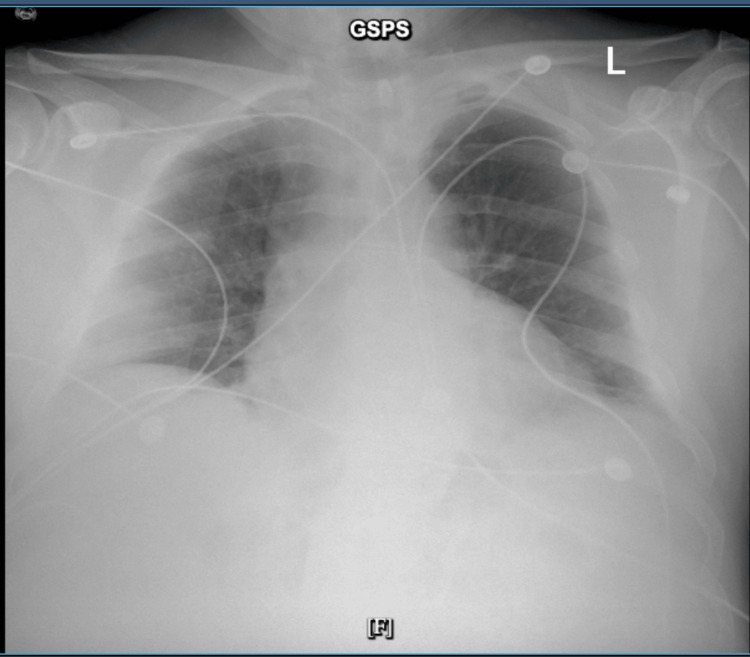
A chest radiograph demonstrated cardiomegaly and left basilar opacities. The image shows an enlarged cardiac silhouette and hazy opacities in the left lower lung zone, compatible with atelectasis or COVID-19-related pneumonitis.
